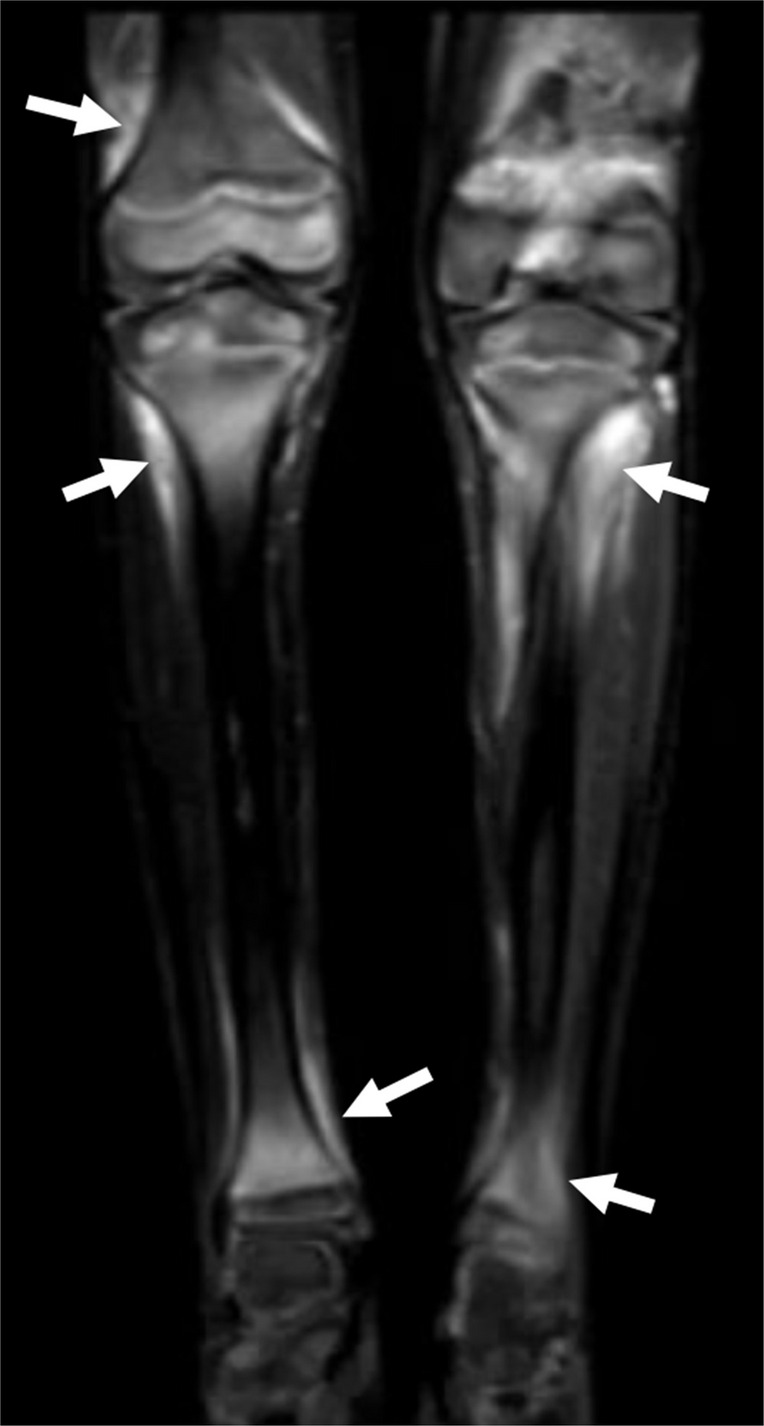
Scurvy. A 4-year-old boy with autism and a very limited diet presented with a limp. Coronal short tau inversion recovery (STIR) MRI of both legs shows bilateral edema-like signal within the distal femurs and proximal and distal tibias. There are adjacent areas of periosteal edema-like signal (*arrows*), which in the setting of scurvy may represent subperiosteal hemorrhage
